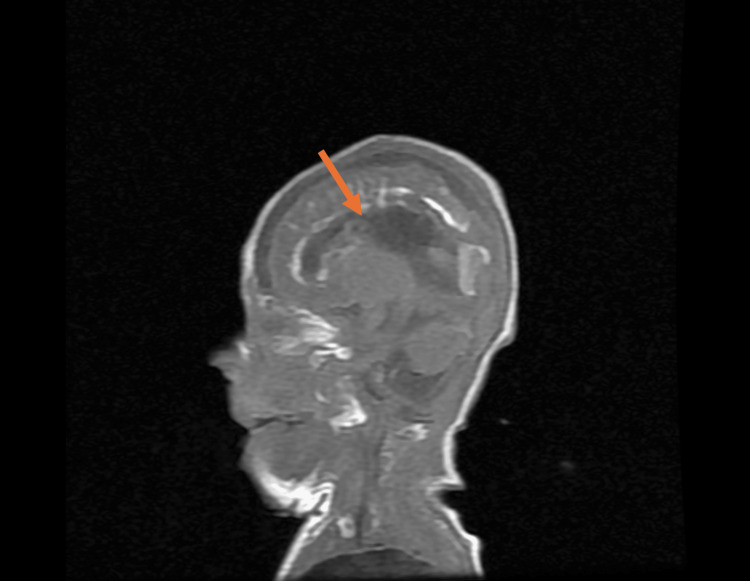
Sagittal plane of brain MRI at day of life 31, displaying agenesis of corpus callosum (orange arrow)
